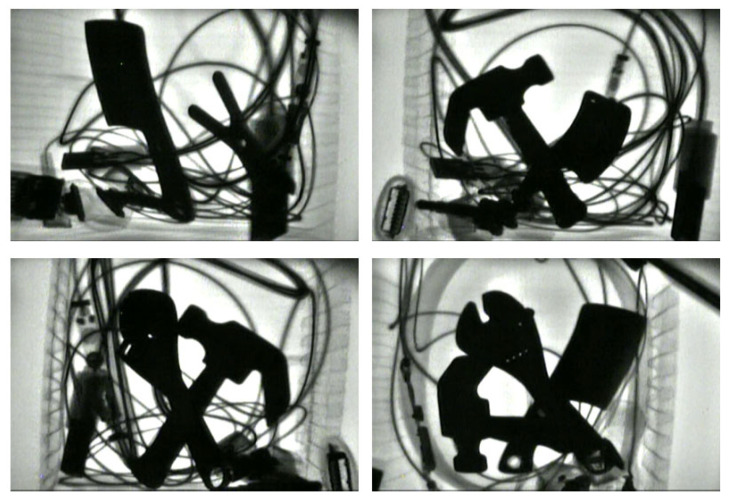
X-ray images of multiple prohibited items with complex background.
